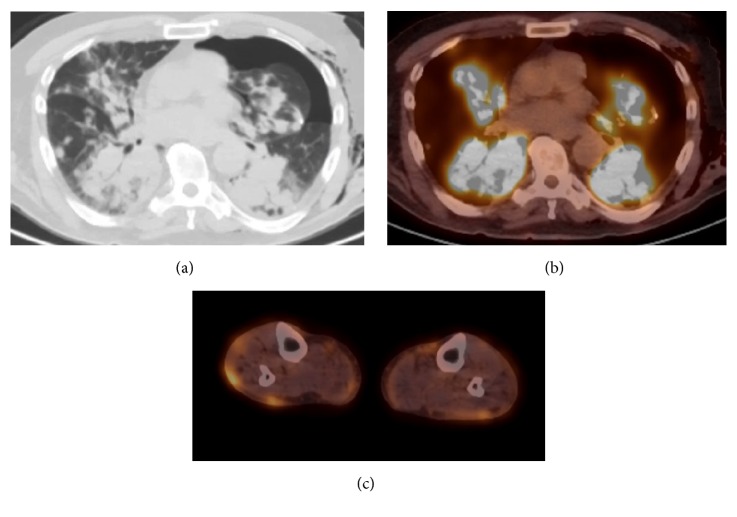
Axial images from PET/CT obtained 4 weeks following baseline chest CT. Axial CT image in lung windows (a) shows marked progression and confluence of bilateral peribronchovascular lung opacities. Fused PET/CT image of the thorax (b) shows the pulmonary abnormalities to have intense FDG uptake with max SUV of 22.3. There were no FDG-avid lymph nodes or splenomegaly. Left pneumothorax and subcutaneous emphysema were a result of the patient's recent VATS biopsy. Axial fused image of the lower extremities (c) shows FDG uptake within multiple subcutaneous nodules.
